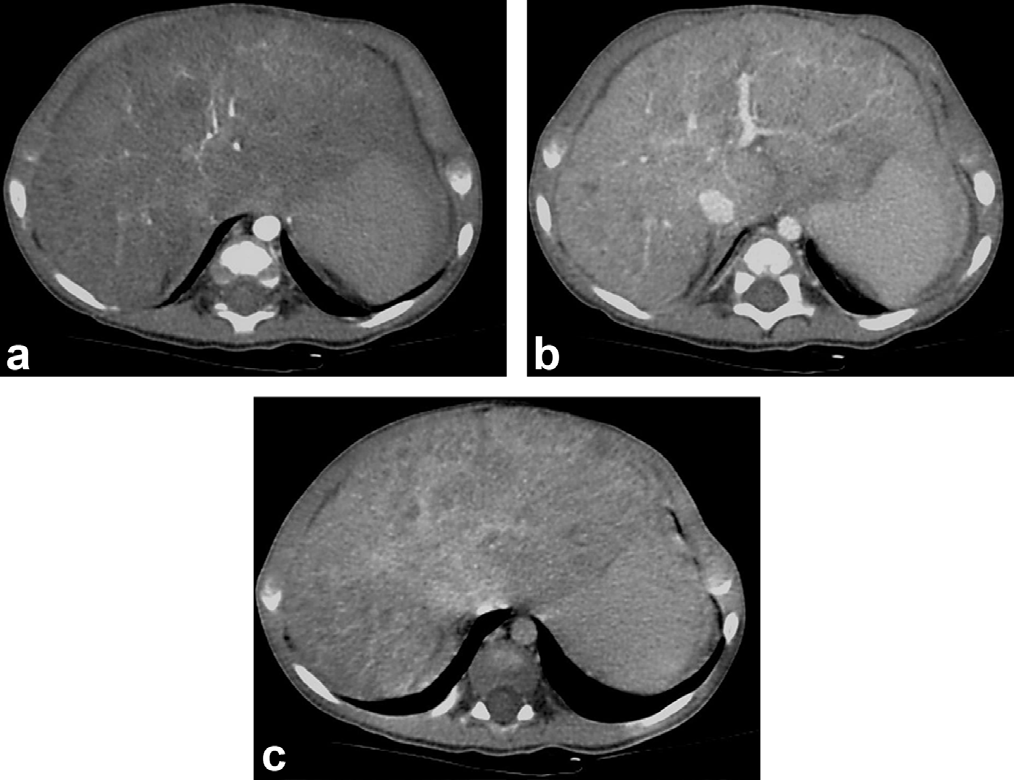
(a) Arterial phase of tri-phasic CT scan shows multiple non-enhancing nodules in liver. (b) Venous phase of tri-phasic CT scan. (c) Delayed images of tri-phasic CT scan.
